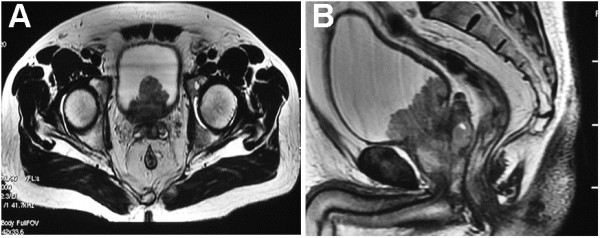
MRI showing of one typical soft tissue. MRI T2-weighted image showing an irregular abnormal signal of soft tissue shadow that intrudes into the bladder on the transverse plane (A). The abnormal signal shadow intruding into bladder is a continuation of the prostate lesion on the sagittal plane (B). The lesion was considered to be PCa with bladder neck involvement.
